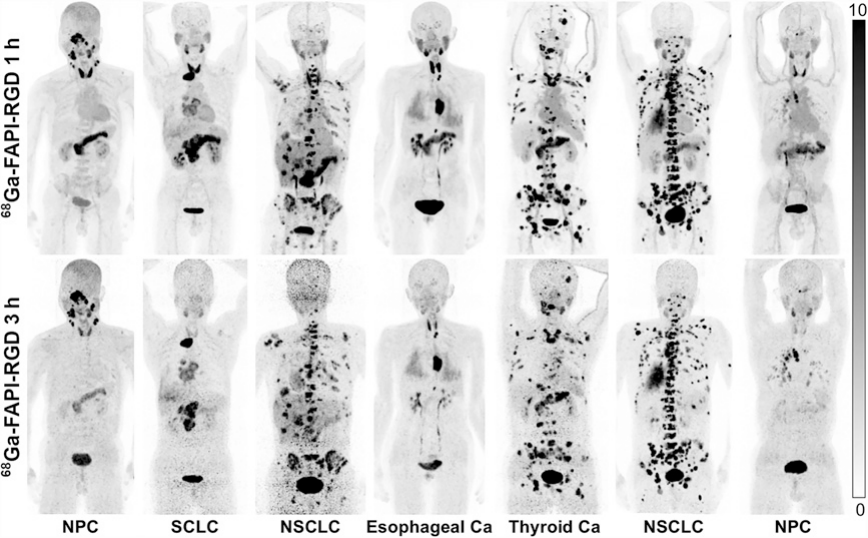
Maximum-intensity-projection images of ^68^Ga-FAPI-RGD PET/CT at 1 and 3 h after injection in different types of cancer. Ca = cancer; NPC = nasopharyngeal carcinoma; SCLC = small cell lung cancer.
